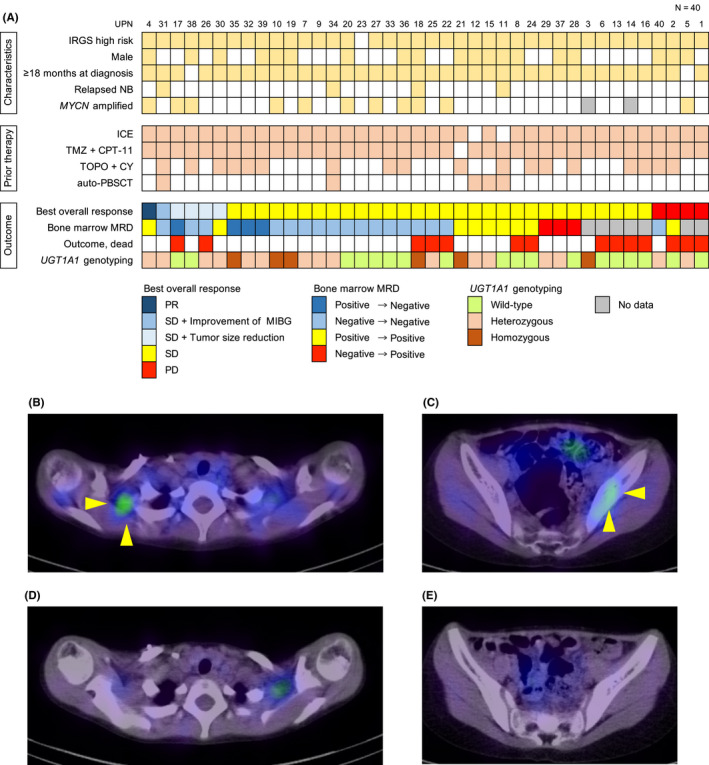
Clinical profiles of patients who received irinotecan, etoposide, and carboplatin (IREC) therapy. (A) Clinical profiles of the 40 patients in this study. Each column indicates 1 patient. (B–E) ^123^I‐MIBG scintigraphy in an 8‐year‐old boy with metastatic neuroblastoma (B, C) before and (D, E) after 3 courses of IREC therapy
